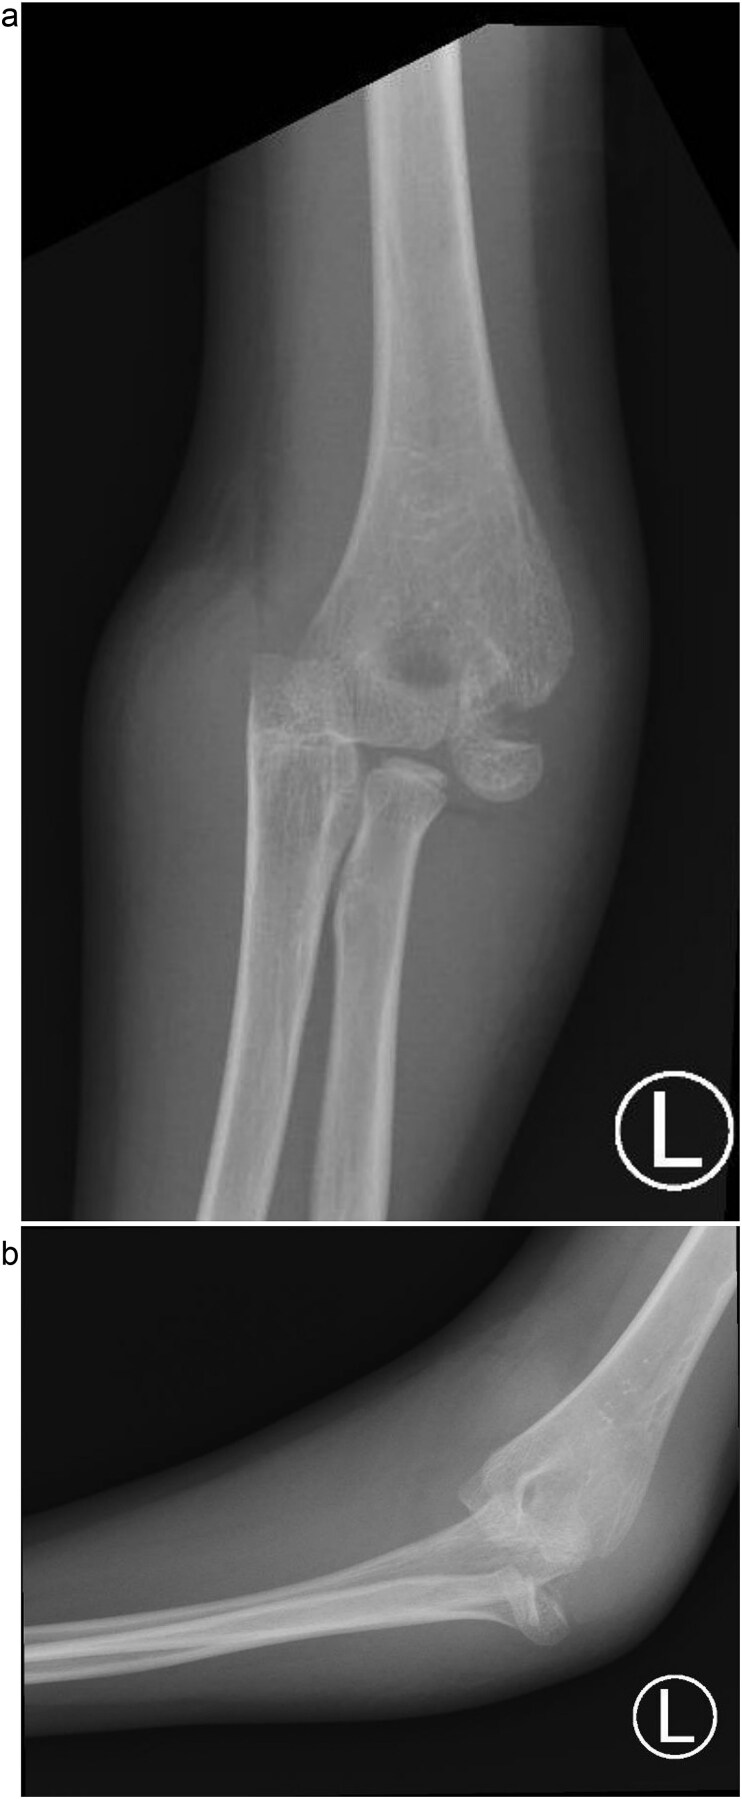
(a) Pre-reduction anteroposterior radiograph of the left elbow showing posteromedial dislocation of the ulnohumeral joint with displaced lateral humeral condyle fracture. (b) Lateral radiograph demonstrating posterior displacement of the olecranon and lateral condyle fragment.
